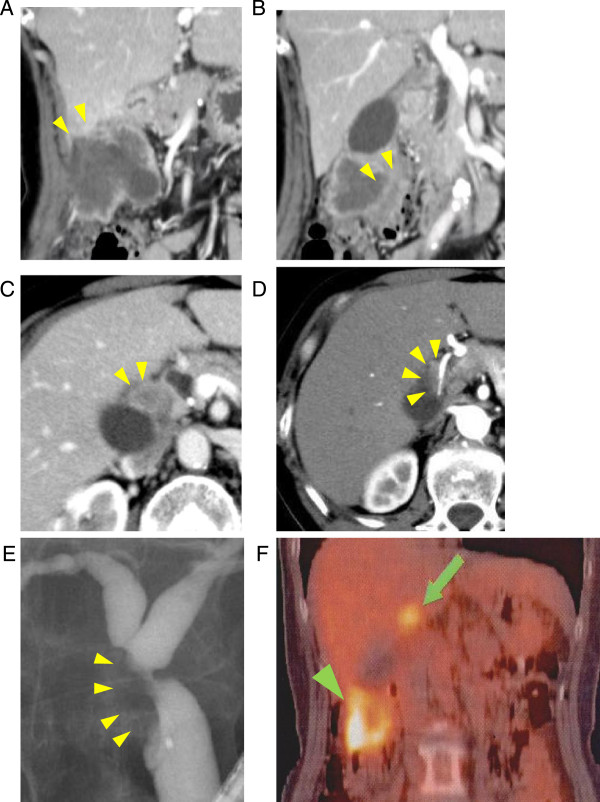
Preoperative imaging studies. (A-D) Contrast-enhanced computed tomography (CT). Arrowheads indicate gallbladder tumor with a peripheral wall enhancement extending to the liver parenchyma adjacent to (A) the gallbladder and (B) the transverse colon. (C) Arrowheads indicate an enlarged lymph node with a diameter of 23 mm, which was detected in the area of the cystic duct. (D) Arrowheads indicate the right hepatic artery (RHA), which runs close to an enlarged lymph node, suggesting that extracapsular invasion extended to the RHA. (E) Endoscopic retrograde cholangiopancreatography: arrowheads indicate a stricture at the level of the junction of the cystic duct and the common hepatic duct. (F) Fluorodeoxyglucose positron emission tomography. The arrowhead and arrow indicate high fluorodeoxyglucose uptake in the gallbladder tumor and cystic duct lymph node, respectively.
